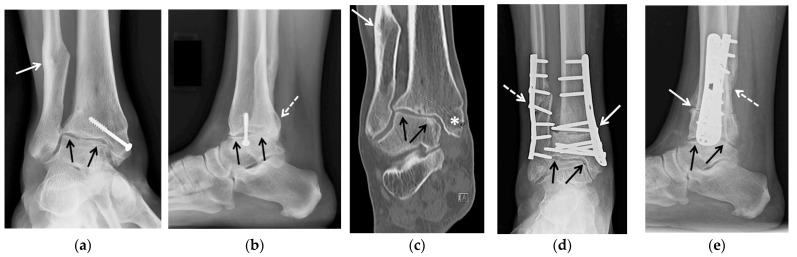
(a–e) A 47-year-old female with a history of remote trimalleolar right ankle fractures and posttraumatic tibiotalar joint osteoarthritis, treated with tibial wedge opening osteotomy and oblique fibular lengthening osteotomy. (a) Mortise and (b) lateral weight-bearing radiographs and (c) coronal reformatted CT image of the right ankle show a healed posttraumatic deformity of the medial malleolus with tibial valgus malunion transfixed by an interfragmentary screw (white asterisk in (c)) and healed posttraumatic deformities of the fibula (white arrow) and of the posterior malleolus (dashed white arrow). Note the asymmetric narrowing of the tibiotalar joint consistent with advanced posttraumatic osteoarthritis (black arrows). (d) Mortise and (e) lateral 3 months postoperative weight-bearing radiographs show improved alignment of the distal tibia (white arrow) and fibula (dashed white arrow) status post osteotomies with decreased tibiotalar joint space narrowing (black arrows). Osteotomy sites demonstrate partial union at 3 months, a normal finding.
